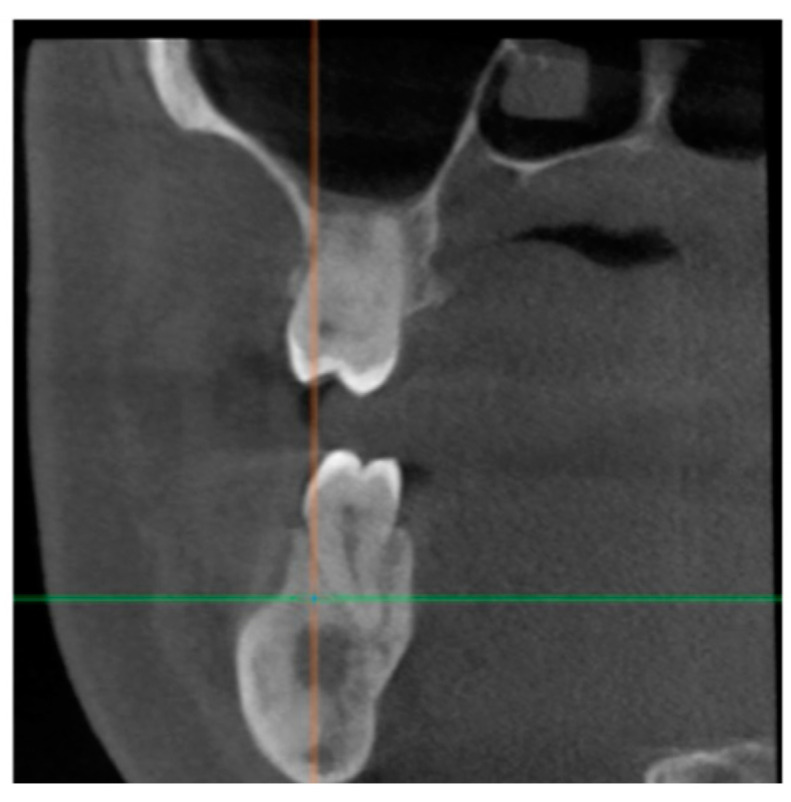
CBCT image of the bone healing process in the tooth #47 periapical area.
